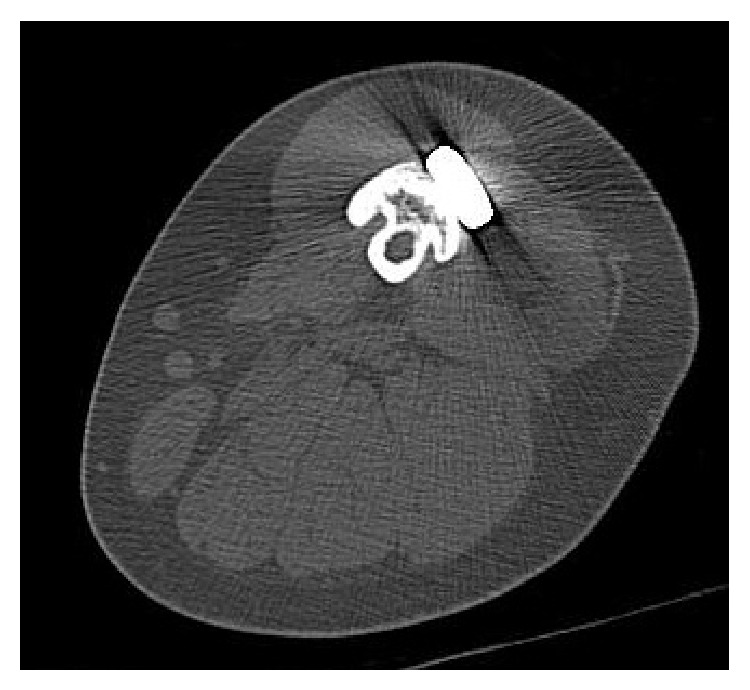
A CT scan at 39 months after surgery. Bridging bone formation from the hypertrophic inlaid fibula to the frozen autograft is evident.
